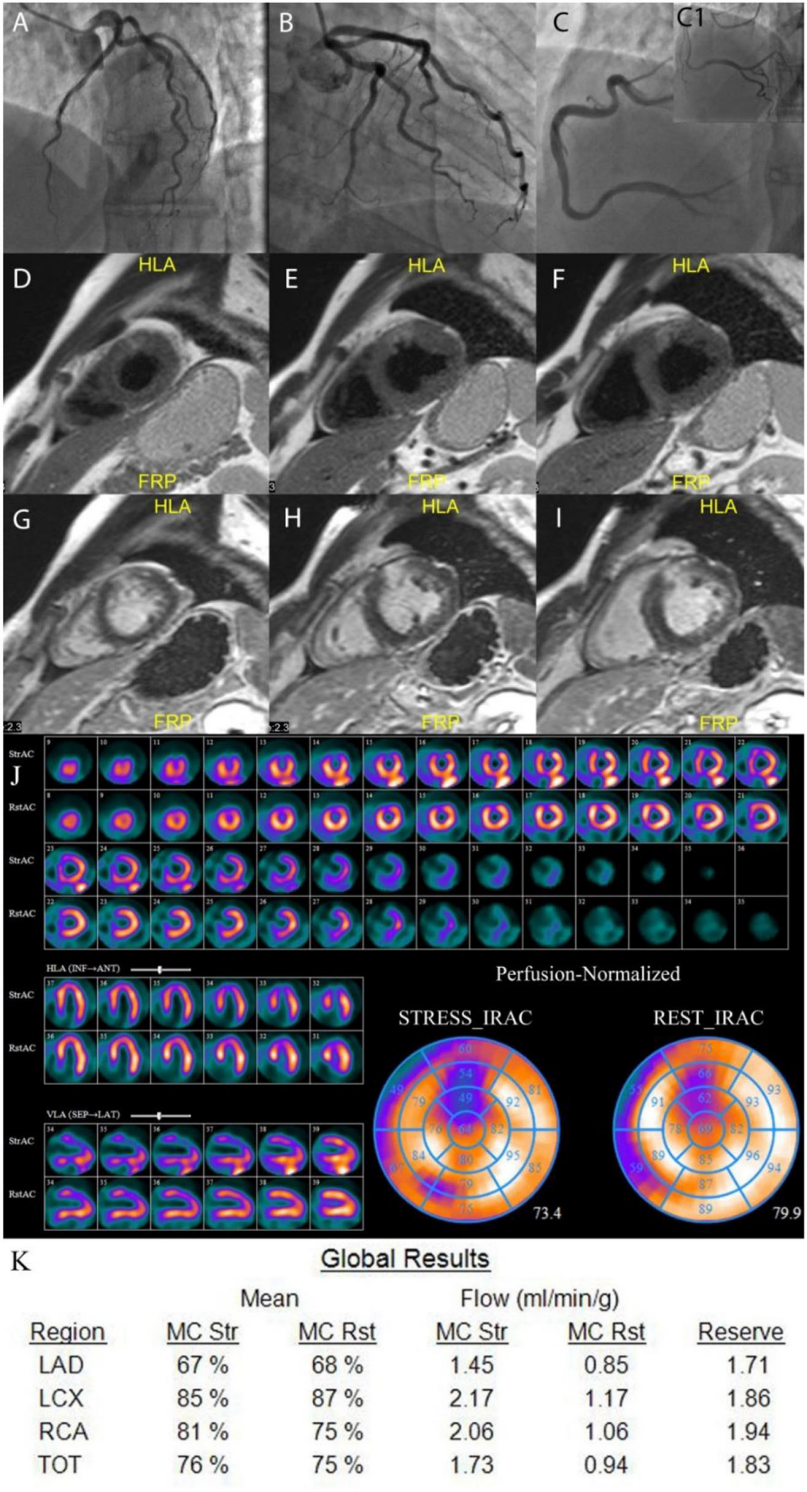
Example of MINOCA patient. A 46 years old male with no obstructive coronary arteries (A–C1). CMR results: T2-weighted images (D–F) show transmural edema in the anterior segments; late gadolinium enhancement images (G–I) show high signal intensity in the same regions indicating necrosis in the anterior segments. The qualitative MPS (J) shows severe moderate to severe partially reversible perfusion defect in the anterior wall. The quantitative CZT SPECT results (K) show abnormal stress MBF and MFR in LAD vessel territory. LAD, left anterior descending artery; LCx, left circumflex artery; RCA, right coronary artery; TOT, total value; MC, motion correction; mL/min/g, milliliters per minute per gram; Rst, rest; Str, stress.
